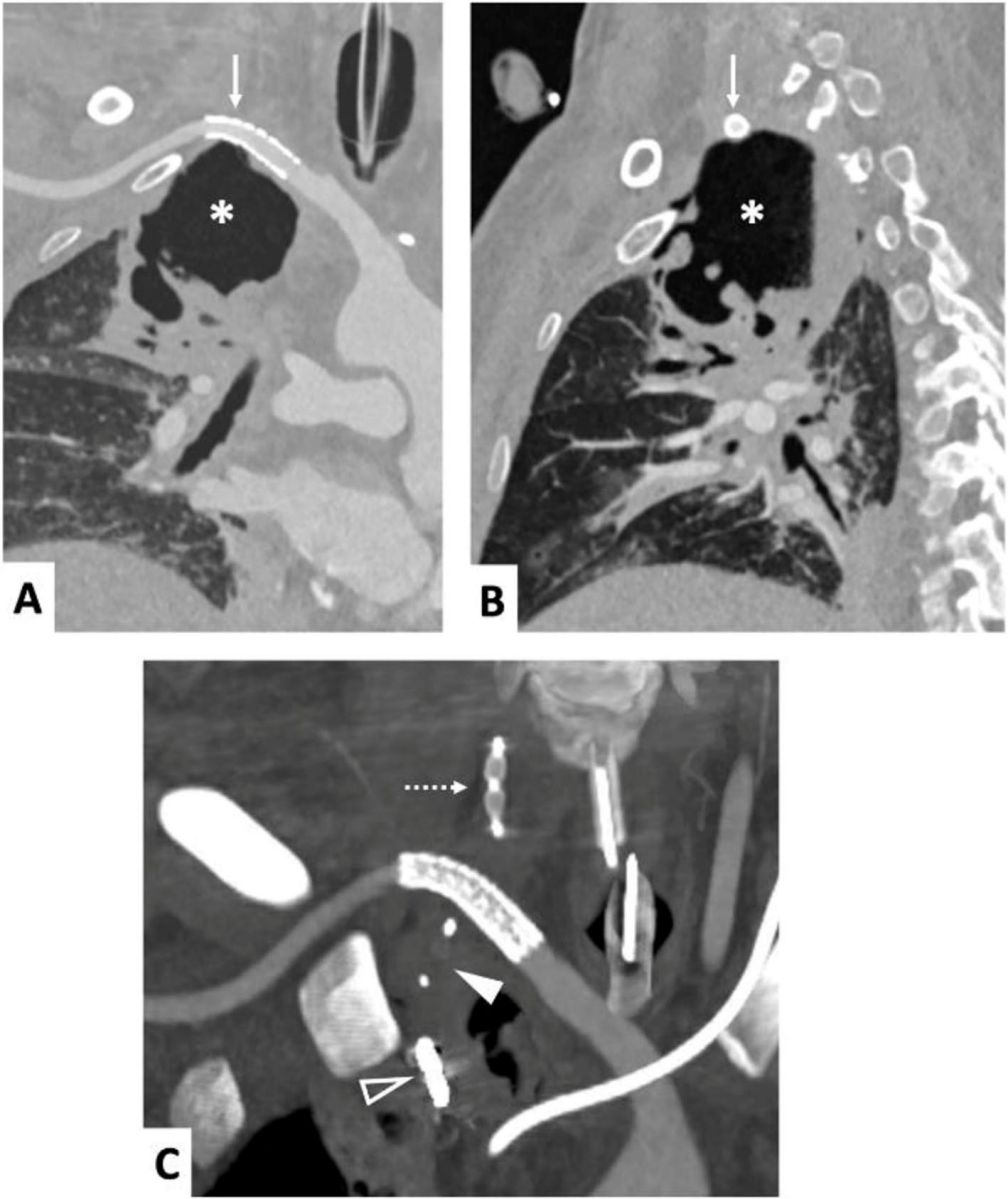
Chest CT-scan in the arterial phase after contrast agent injection, coronal (3-A and 3-C), sagittal view (3-B). Right SCA with regular caliber and patency of the stent graft (3-A and 3-B, arrow), adjacent to the upper pole of the right apical pulmonary excavation where the pseudoaneurysm was located (3-A and 3-B, asterisk). Occlusion of the proximal segments of the right ITA with a microvascular plug (3-C, solid arrowhead) and coils (3-C, hollow arrowhead), and of the right VA (3-C, dotted arrow)
